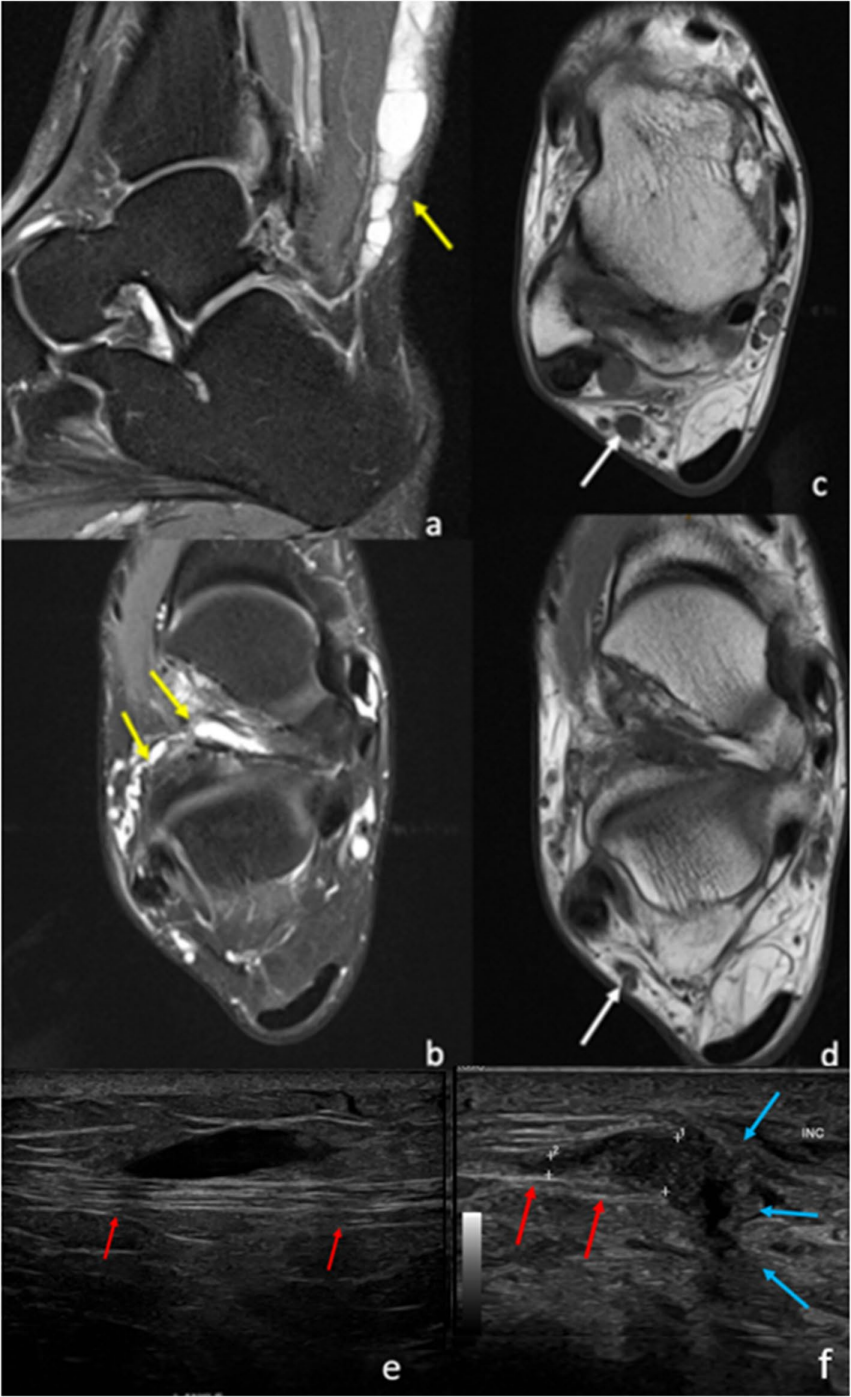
25-year-old man with sural nerve symptoms and numbness. Sagittal (a) and axial (b) PDFS and axial PD (c and d) demonstrate a ganglion (yellow arrow) arising from the sinus tarsi with superior and intraneural extension extending into the calf along the path of the sural nerve (white arrow). e, f Ultrasound images of a different patient, a 45-year-old female with neuropathic pain in the lateral foot following subtalar joint fusion from post-traumatic osteoarthritis. e The normal fibrillary structure of a normal segment of proximal sural nerve (red arrows). More inferiorly, at level of the surgery (f), there is hypoechoic enlargement of the sural nerve as it traverses the scar tissue (blue arrows), consistent with sural nerve entrapment
